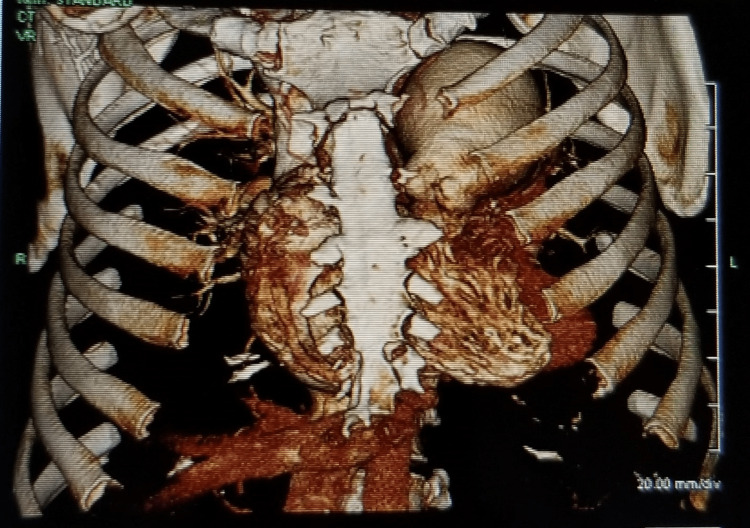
3D reconstruction of CTA showing pulmonary artery aneurysm
